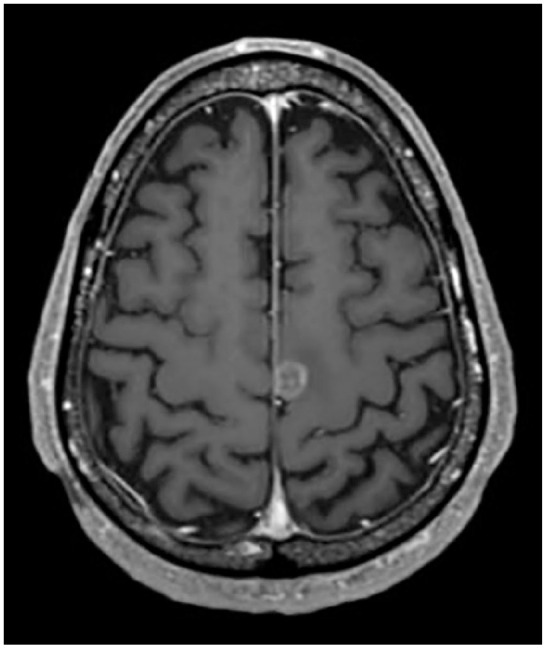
An exemplary magnetic resonance image of a patient treated for a single brain metastasis.
